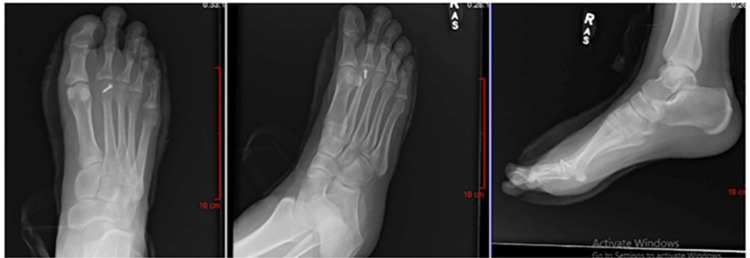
Example of mock patient scenario (Case 1) provided to surgeons; Post-operative radiographs demonstrating open reduction and metatarsal shortening (Weil) osteotomy.
